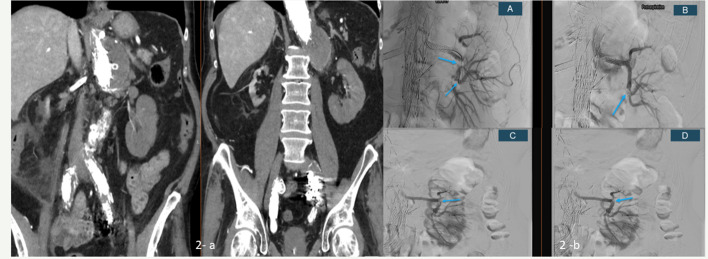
A 65-year-old patient with a thoracoabdominal aortic aneurysm treated with BEVAR. Baseline eGFR was > 90 mL/min. Two months after BEVAR, the patient was admitted to another hospital with anuria and back pain. The patient was transferred to our hospital more than 24 h after symptom onset with an eGFR of 9 ml/min. Contrast-enhanced computed tomography with coronal reconstruction showed occluded bilateral renal stent grafts and atrophy of the right kidney (a). b Recanalisation of the left renal stent graft and relining with a Viabahn stent graft, with a successful outcome despite some residual thrombus (A-D-arrows). The patient is not on dialysis, and the eGFR is 37 mL/min
